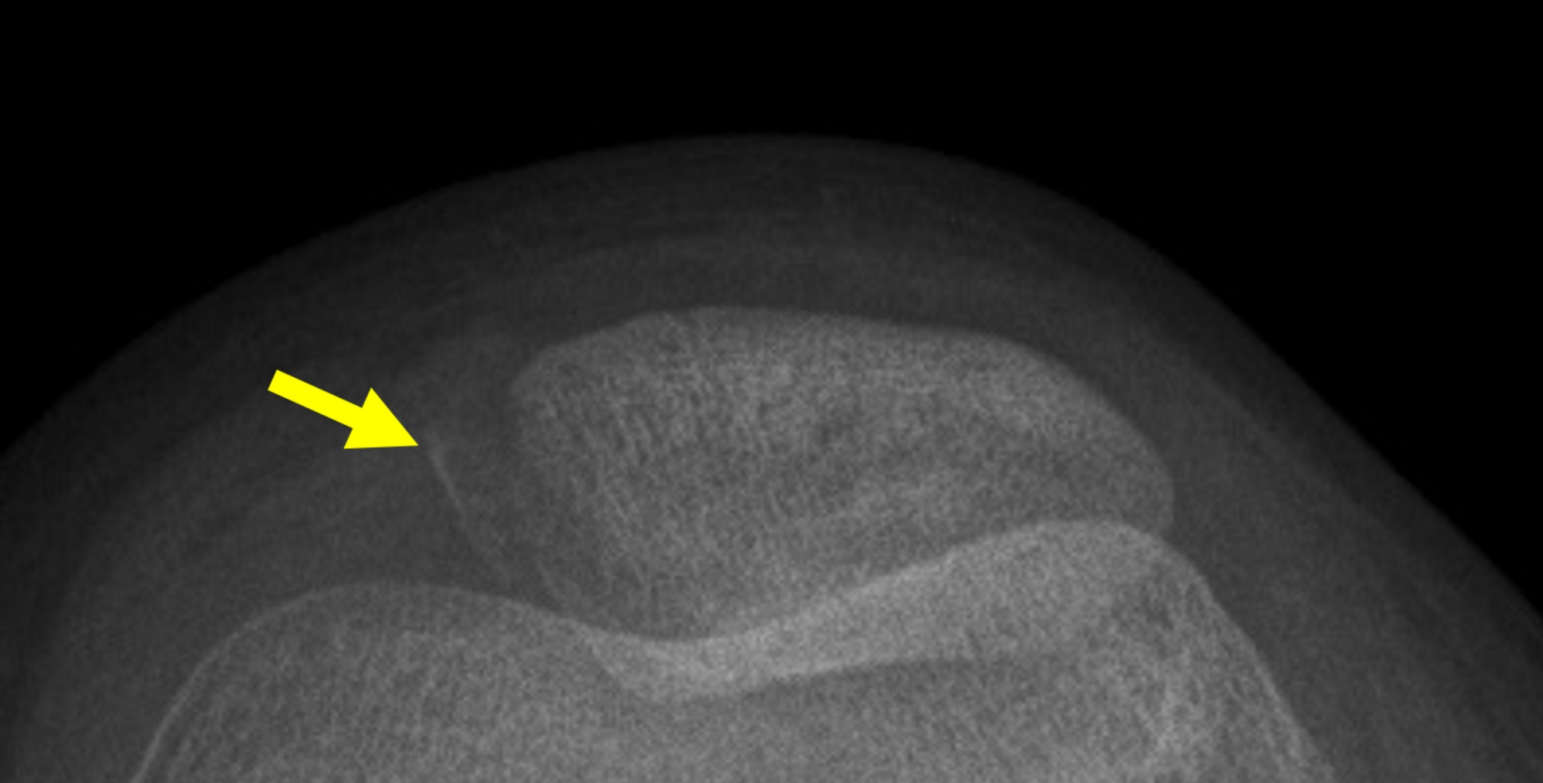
Axial X-ray of the left patella Arrow indicates avulsion fracture of the medial patellar border
